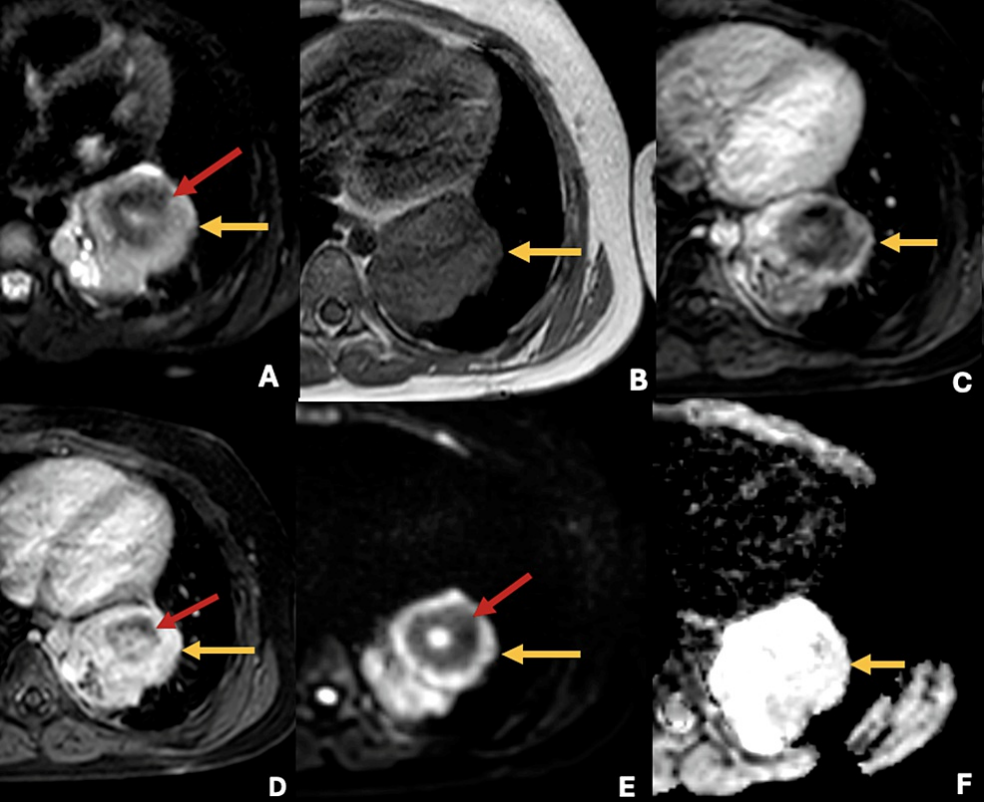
Thoracic MRI shows an intrapulmonary mass with high signal intensity in T2 sequences (yellow arrow in A), low signal intensity in T1 sequences (yellow arrow in B), with late contrast enhancement (yellow arrows in C and D), and no diffuse restriction (yellow arrows in E and F); central calcifications are shown (red arrows).
